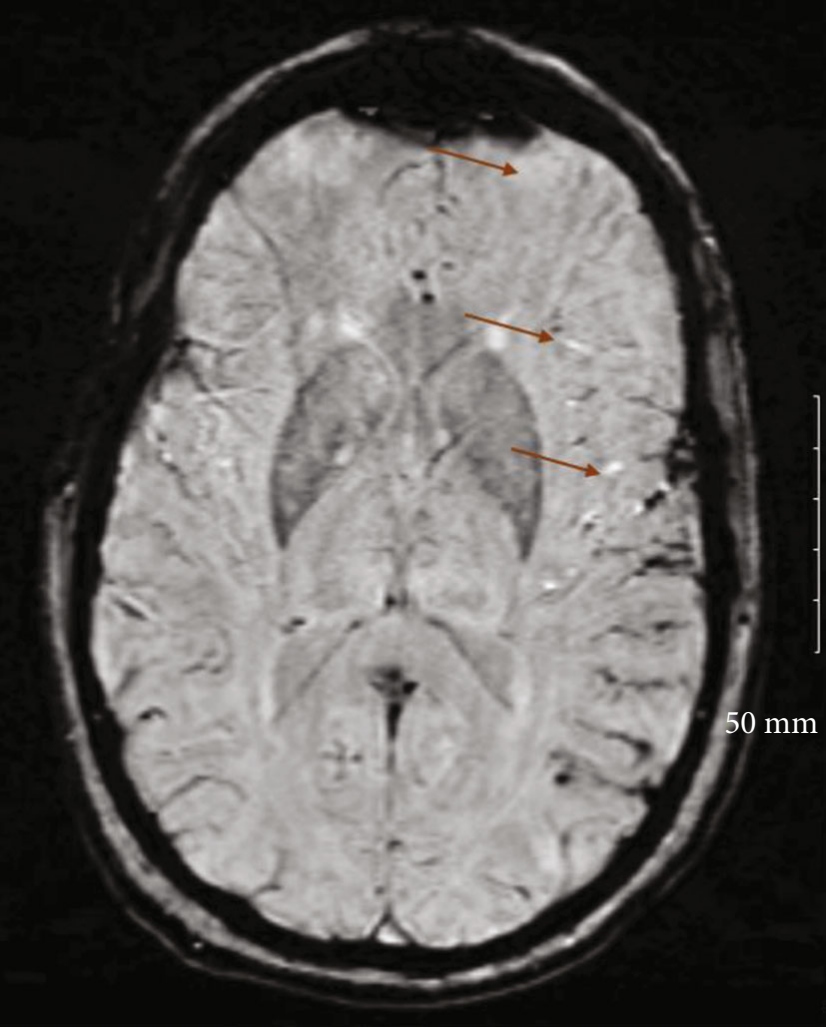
Susceptibility weighted magnetic resonance imaging showing the multifocal left frontal and parietal cortical infarction.
